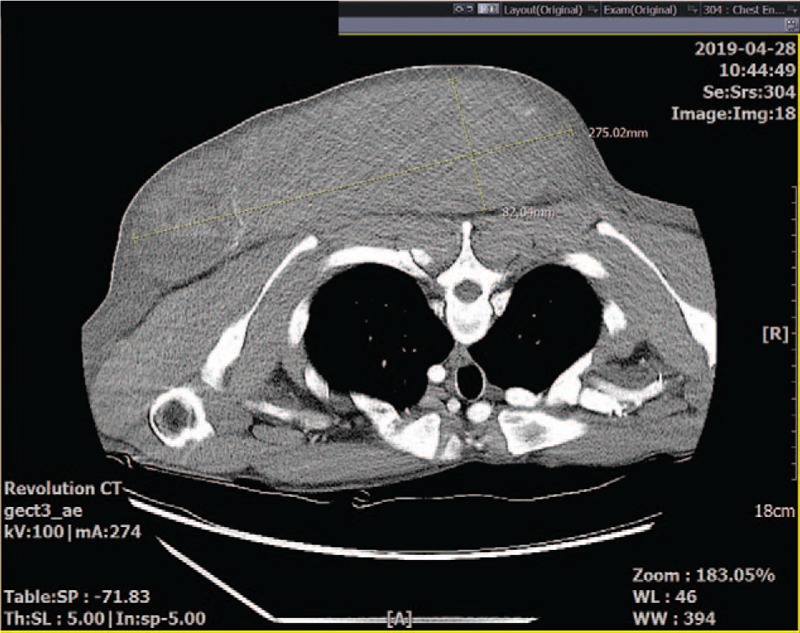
Preoperative chest computed tomography depicting a huge mass of 28 cm in width and 8 cm in height in the left upper back.
